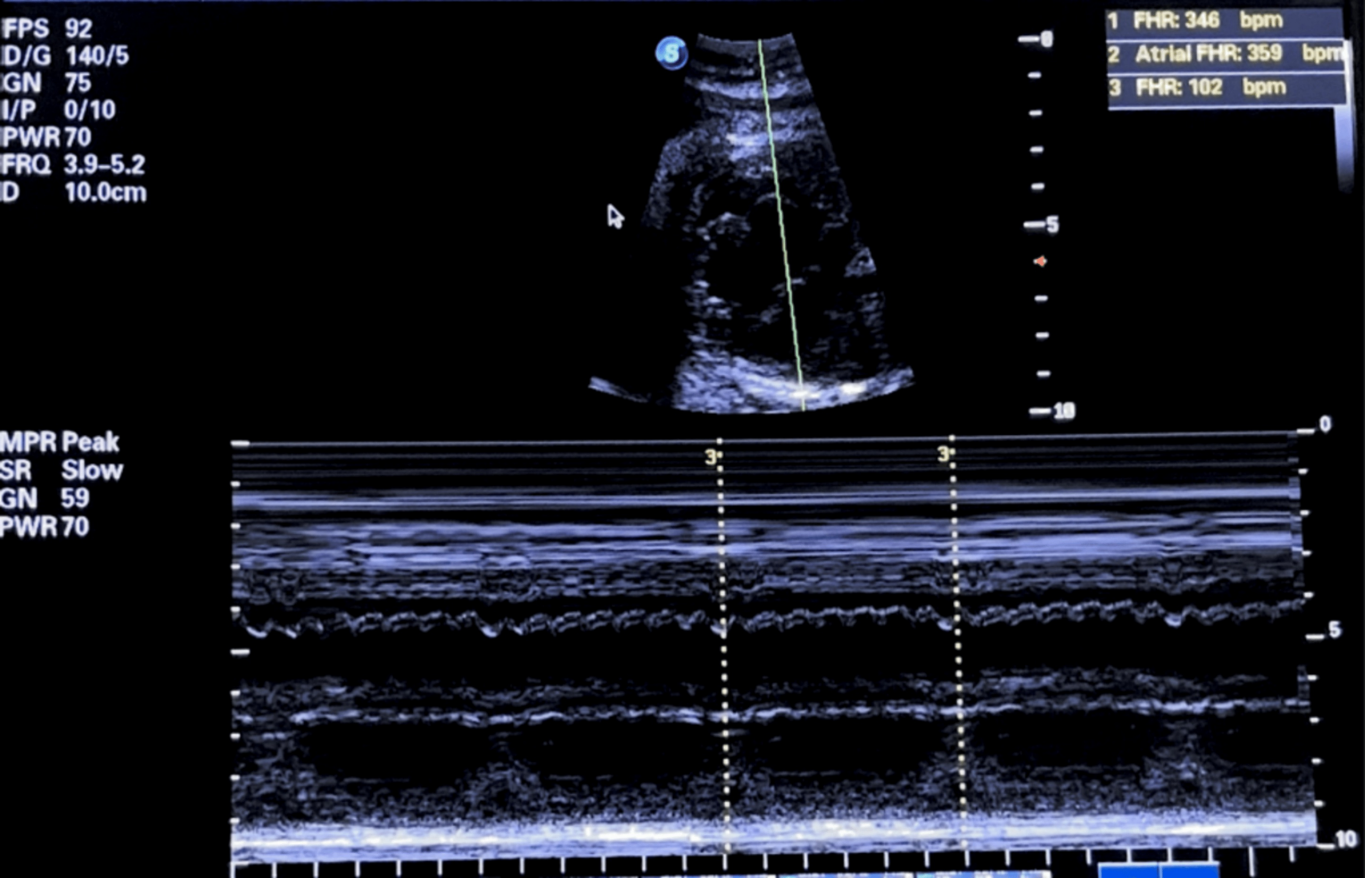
Fetal M-mode showing severe atrioventricular dissociation with bradycardia M-mode echocardiography shows complete AV dissociation: atrial rate: 359 bpm, ventricular rate: 102 bpm (ratio 3:1), confirming third-degree AV block with complete absence of AV conduction.
